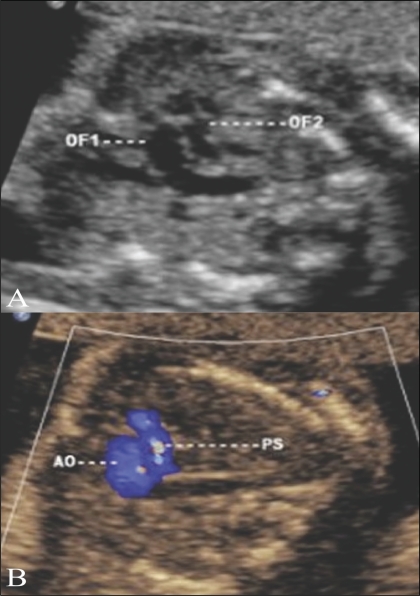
Transposition of the great arteries. An image through both outflow tracts (A) shows the two outflow tracts parallel to each other (OF1, OF2). The color Doppler image (B) shows that the flow in both outflow tracts is in the same direction. Ao – aorta, PS – pulmonary artery.
